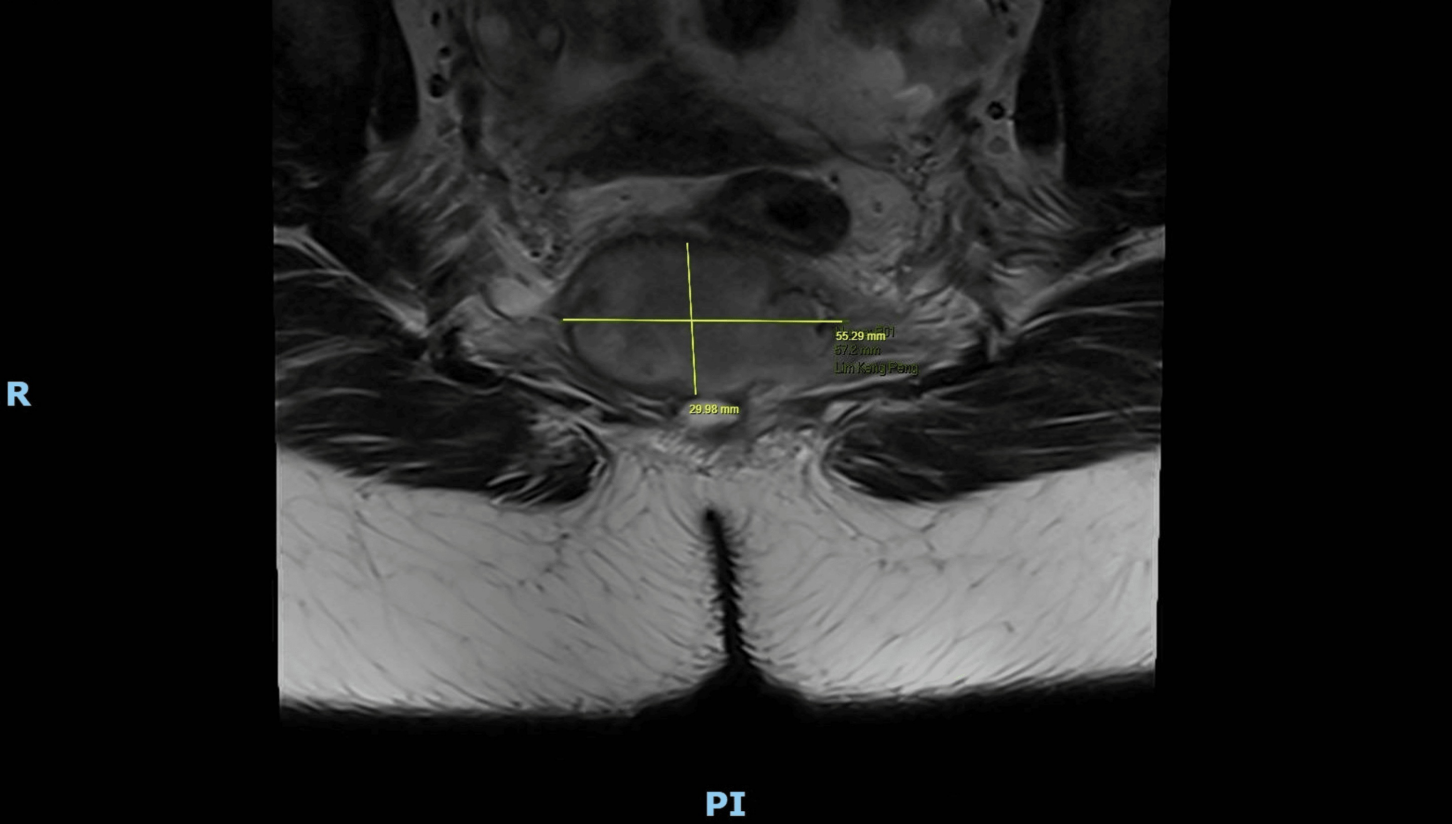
Axial MRI pelvis (2025) highlighting lobulated lesion with restricted diffusion and peripheral enhancement extending into surrounding soft tissue
